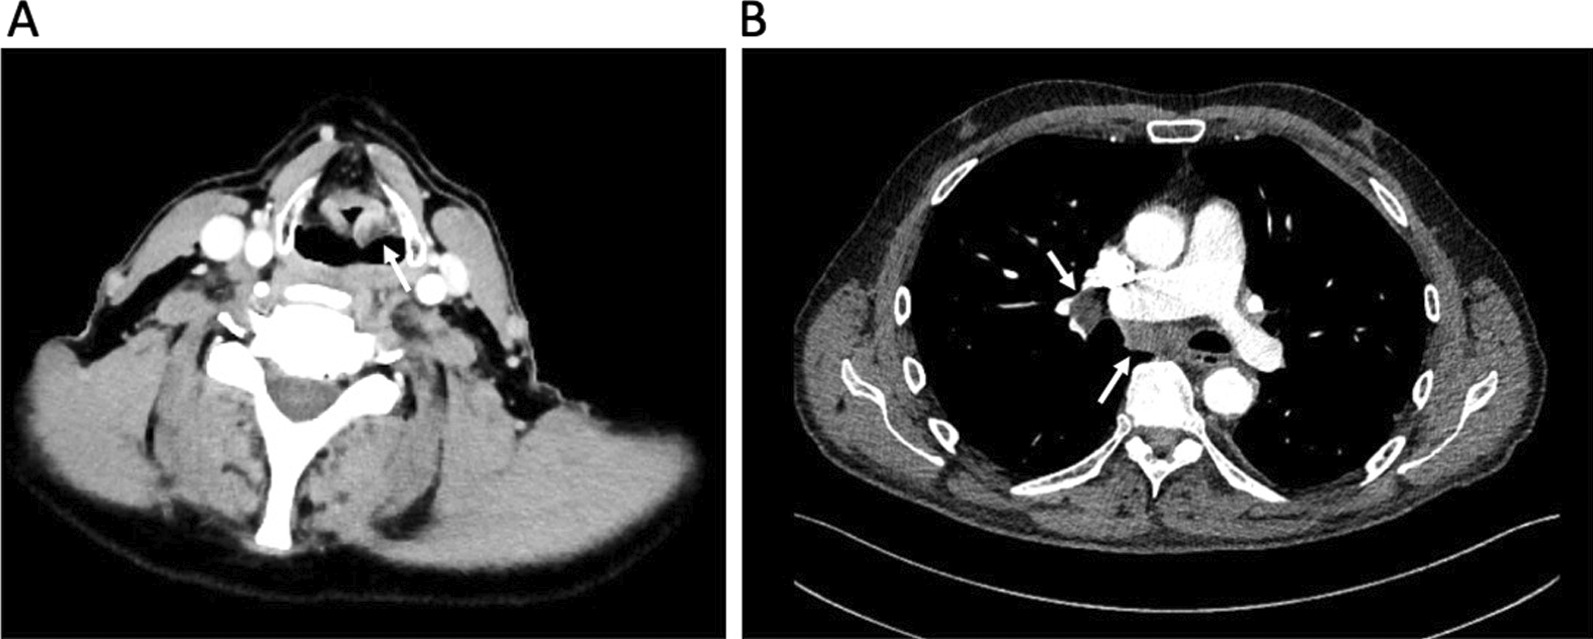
CT scans of the laryngeal cancer (A) and lung adenocarcinoma (B) before treatment start. A Transversal CT images of the neck showing a moderately contrast enhancing tumor of the left vestibular fold measuring 12 × 13 × 11 mm (white arrow). B Transversal CT scan of the thorax showing mediastinal and right hilar nodal metastases (white arrow)
